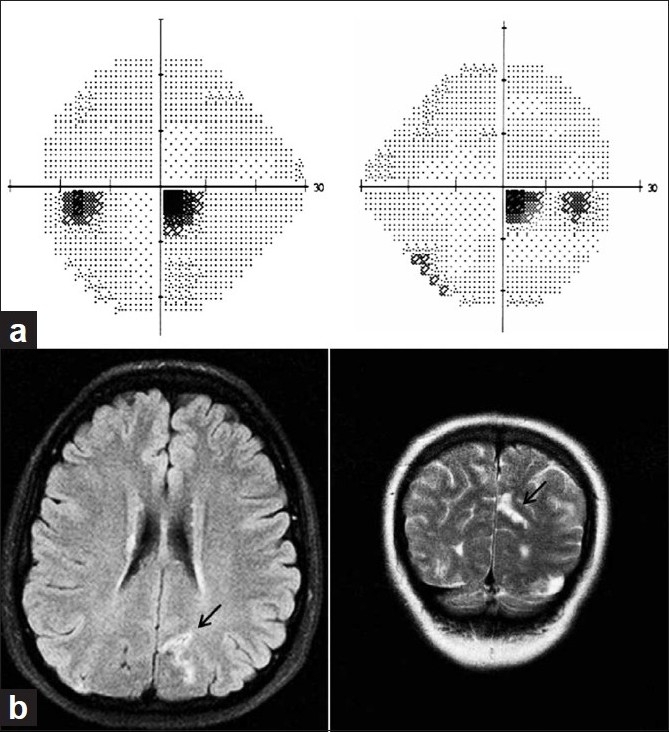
(a) Visual fields of a 38-year-old who complained of severe difficulty reading, shows a right inferior homonymous scotoma. (b) MRI brain of the same patient shows a lesion in the superior bank of the left calcarine cortex
